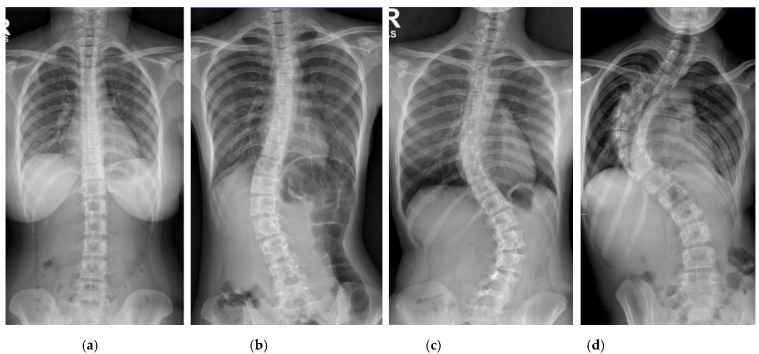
Examples of standard X-ray images exported from the PACS: (a) CA < 10°; (b) CA 10° to 25°; (c) CA > 25° to 40°; (d) CA > 40°.
